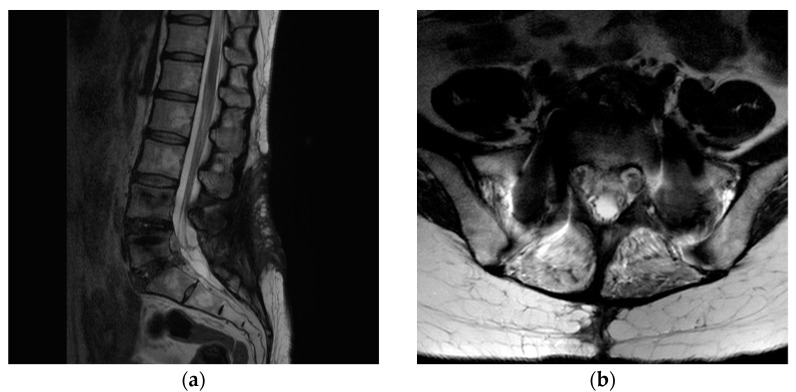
(a,b) Magnetic resonance imaging at 6 months postoperatively showed a well-decompressed thecal sac and nerve roots. However, roots were clumped and distorted within the thecal sac, suggesting arachnoiditis.
